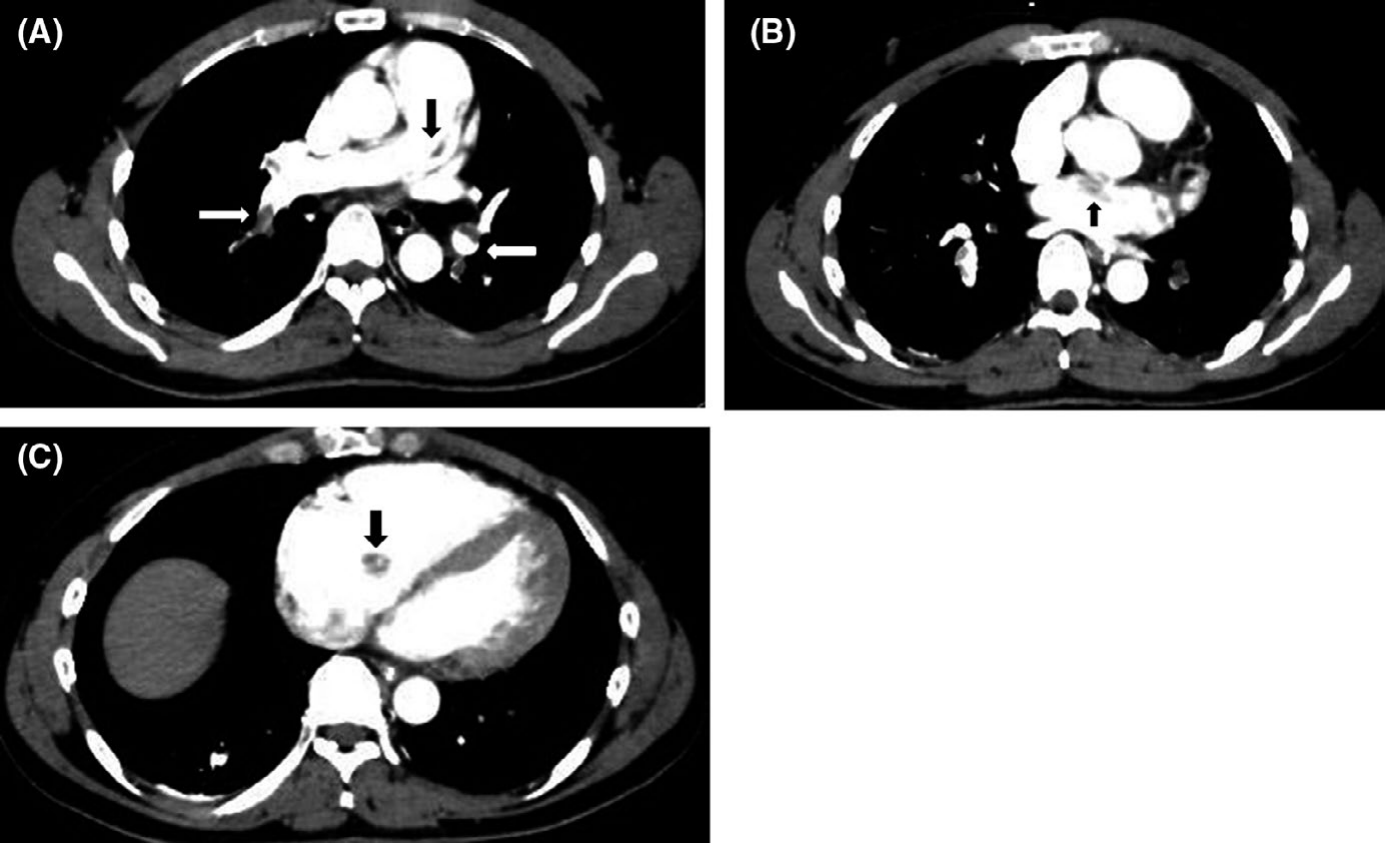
Preoperative chest computed tomography scan showing massive pulmonary emboli (A) and thrombi in the left (B) and right (C) atria (arrows)
